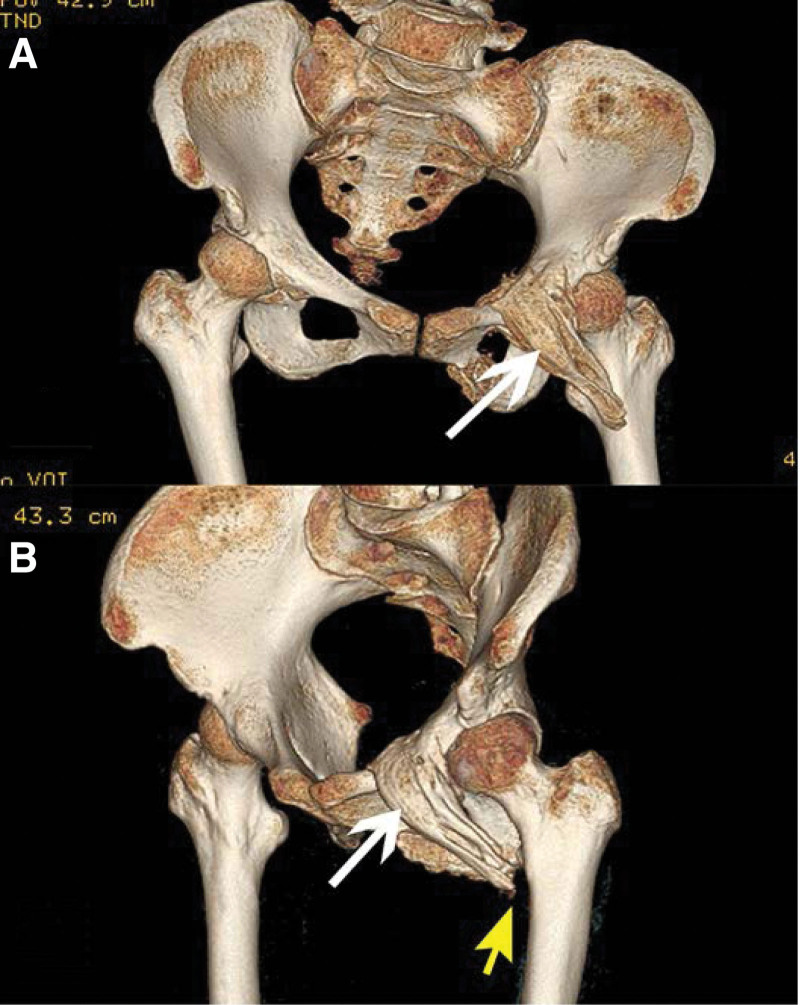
The 3-dimensional reconstruction CT views taken 4 mo after the accident. (A) Is the front view and (B) is the left oblique view. The views clearly show an osseous muscle-shaped lesion located between the superior pubic ramus to the lesser trochanter (white arrows) and a narrow space between the lesion and the lesser trochanter (yellow arrow). This suggested that the lesion may not be attached tightly to the femur. CT = computed tomography.
